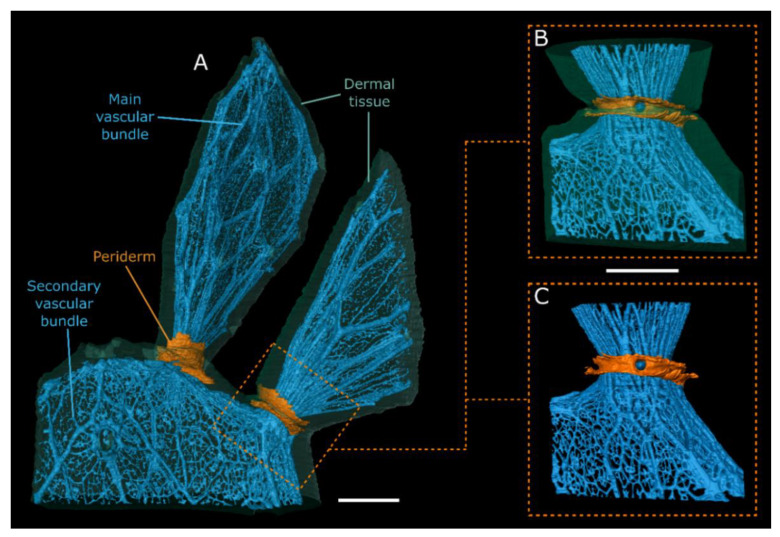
MRI scans of *Opuntia ficus-indica*. 3D representation of the dermal tissue ((A,B); transparent green), the vascular system ((A–C); blue) and the peridermal tissue ((A–C); orange) of a junction site and parts of the connected lateral and sub-lateral branches, based on the segmentation of MRI scans. (A) Visualisation (lateral view) of an overview scan (sample 1). Please note that the right and lower branch had to be trimmed because of space limitations of the MRI scanner. Visualisation (lateral view) of the surface coil scan (sample 2) with (B) and without (C) representation of dermal tissue. All scale bars equal 1 cm.
